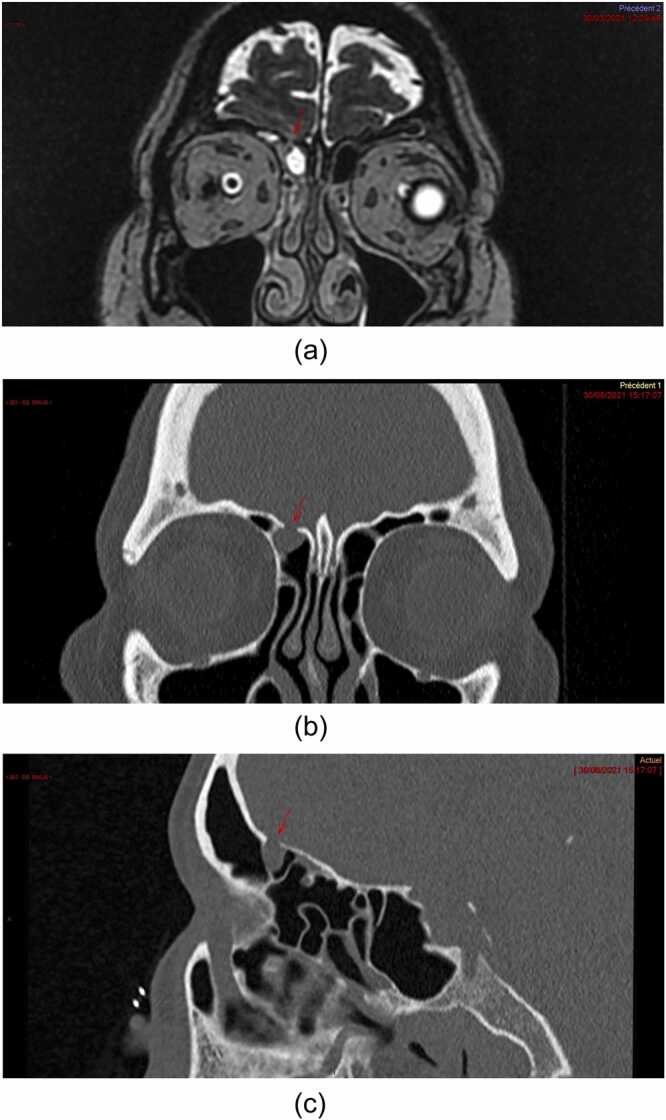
Imaging after the CSF leak. A. MRI, coronal view: head MRI in the coronal plane (T2 sequence) demonstrating a meningocele (arrowhead) situated under the right ethmoid. B. CT, coronal view: brain CT image in the coronal view demonstrating the right ethmoid breach (arrowhead). C. sagittal view: brain CT image in the sagittal view demonstrating the breach and the meningocele (arrowhead). CSF, cerebrospinal fluid; CT, computed tomography; MRI, magnetic resonance imaging.
